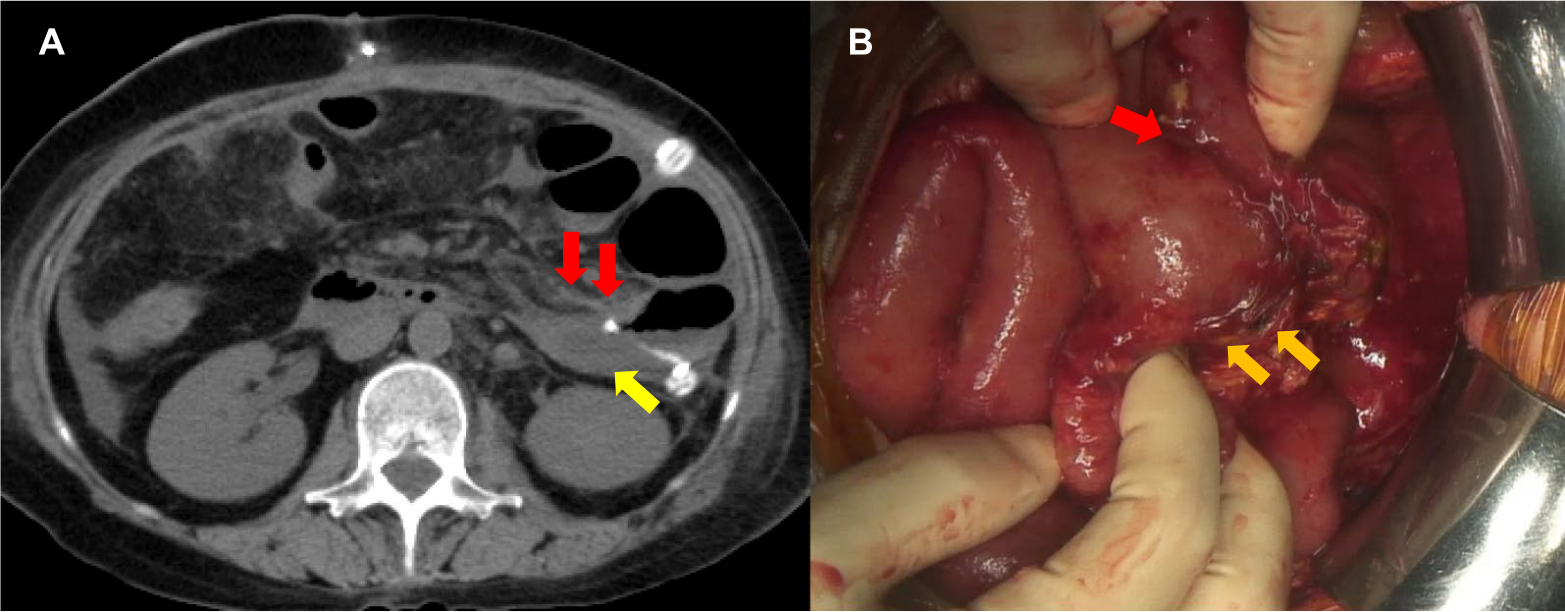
A Abdominal computed tomography showing fluid collection (yellow arrow) near the jejunojejunal anastomosis (red arrows). B Intraoperative image showing dehiscence at the entry hole of the linear stapler (orange arrows) near the jejunojejunal anastomosis (red arrow)
